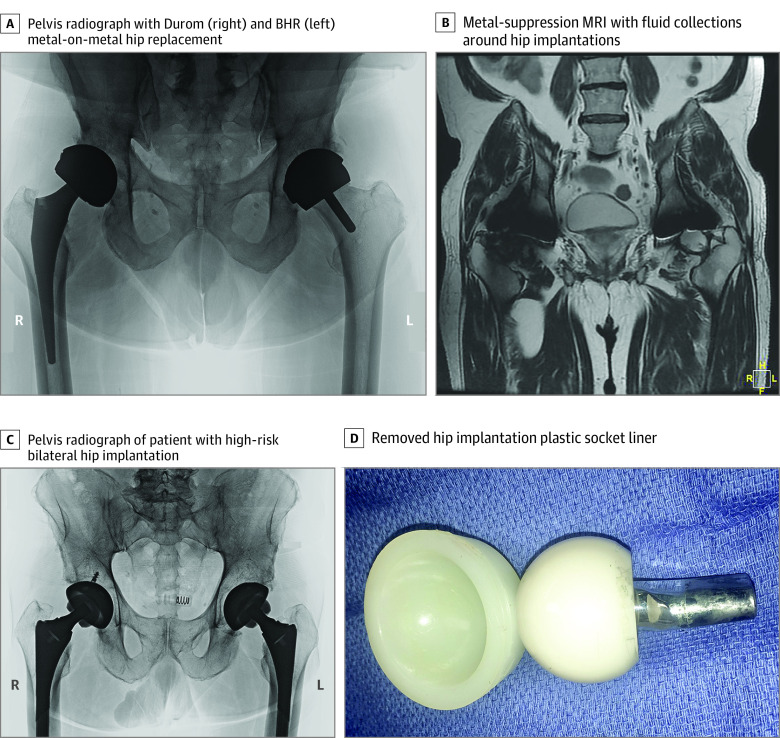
Images of Metal-on-Metal and Modular-Neck Hip Implantations A, The patient with an extreme-risk right metal-on-metal hip replacement implanted in 2007 and a left metal-on-metal hip resurfacing implanted in 2009 presented complaints of tremor, fatigue and forgetfulness, and hip swelling and stiffness. Cobalt levels in urine were 23.5 ppb; in blood, 5.1 ppb; in joint fluid, 440 ppb. Focal brain hypometabolism consistent with toxic encephalopathy was noted on fluorodeoxyglucose-positron emission tomography brain scan. B, Metal-suppression magnetic resonance imaging (MRI) of same patient shows abnormal fluid collections around the medial subtrochanteric area of the right femur and in the joint space of the left hip. C, Patient with high-risk bilateral hips (Microport) with modular cobalt-chrome femoral necks and alumina ceramic femoral heads implanted in 2013, presented with complaints of tremor, fatigue, anxiety, and groin pain. Cobalt levels in urine were 21 ppb; in blood, 4.1 ppb; in joint fluid, greater than 1000 ppb. Focal brain hypometabolism was consistent with toxic encephalopathy noted on fluorodeoxyglucose-positron emission tomography brain scan. D, The explanted left hip plastic socket liner has an alumina ceramic head and a modular cobalt-chrome femoral neck. Note extensive corrosive debris on distal male end of neck.
